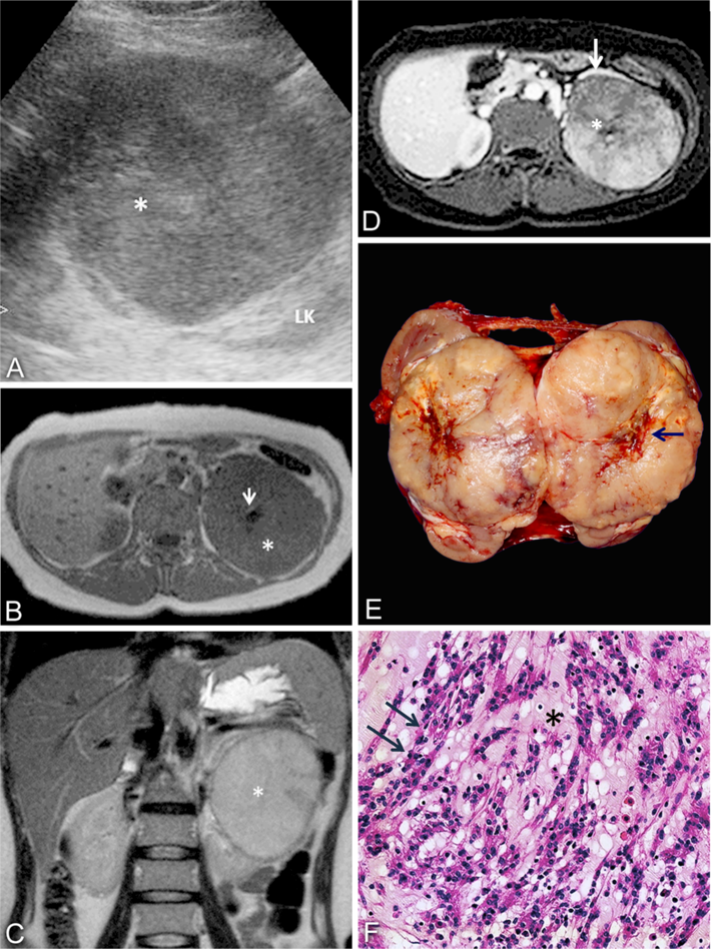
Imaging findings. (A) Ultrasound image showing a heterogeneous mass (*) in the upper pole of the left kidney (LK). (B) Axial T1-weighted and (C) coronal T2-weighted imaging demonstrating a mass in the left kidney (*), with homogeneous low T1 and a high signal in T2. A central scar (arrow) is seen on axial T1. (D) Axial T1, postcontrast, showing a hypovascular lesion compared to renal cortex (white arrow) with a central scar (*). (E) Gross findings: well-circumscribed tumor with a central scar (arrow). (F) Elongated tubules and cord arrangements (arrows) embedded in a mucinous stroma (*). LK, left kidney.
